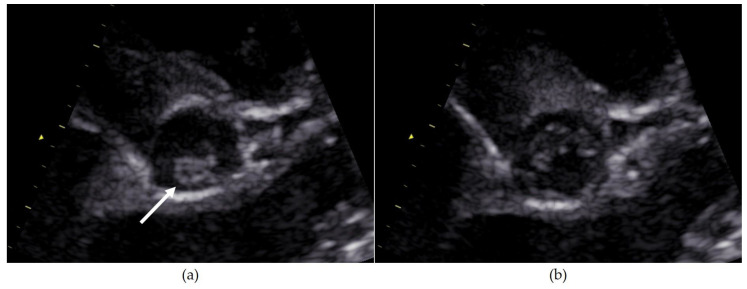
Echocardiographic images of a unicuspid valve. (a) Single commissural attachment zone (arrow). (b) Eccentric, “pinhole-like” valvular opening during systole. The margin opposite the commissural attachment is rounded.
